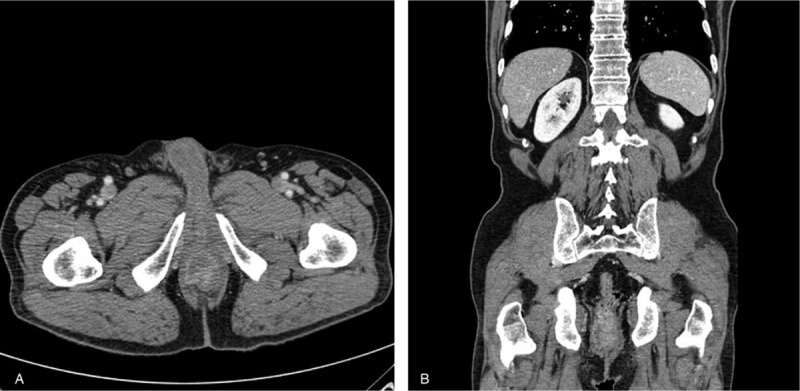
Tumoral infiltration of the rectal cancer on abdominopelvic computed tomography scan. (A) Axial image. (B) Coronal image.
